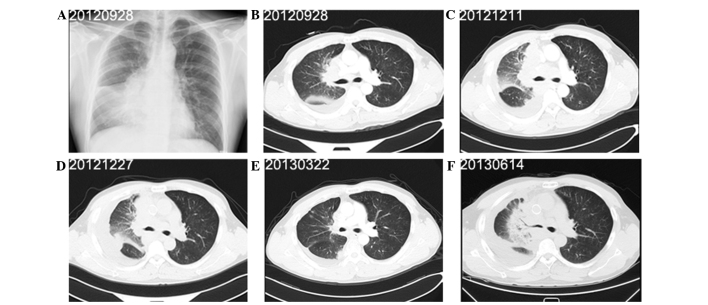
Case three: Representative images from radiography and chest CT revealing the presence of a mass in the middle lobe of the right lung, pleural effusion and enlarged lymph nodes. (A) Radiograph prior to treatment. (B) Representative CT image prior to treatment. (C) Representative CT image after two cycles of first-line chemotherapy. (D) Representative CT image after superior vena cava stenting. (E) Representative CT image after thoracic radiation therapy and four cycles of second-line chemotherapy. (F) Representative CT image showing progressive disease after second-line chemotherapy. CT, computed tomography.
